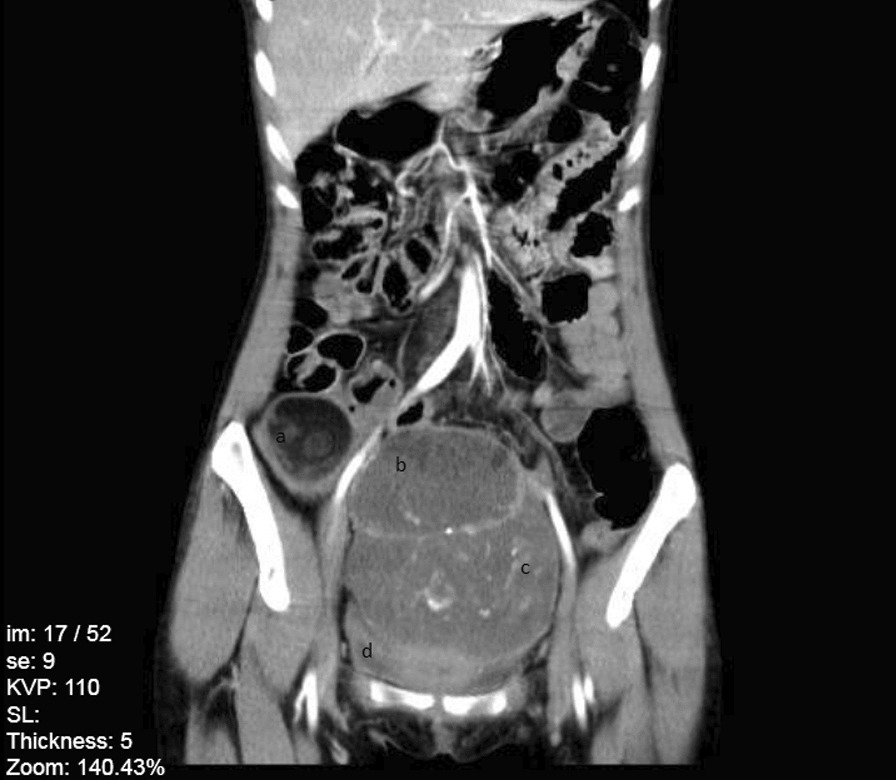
Abdominal CT-Scan with intravenous (IV) contrast, coronal view. a Right ovarian mass, b Uterine, c Left Ovarian mass, d Bladder (empty)
